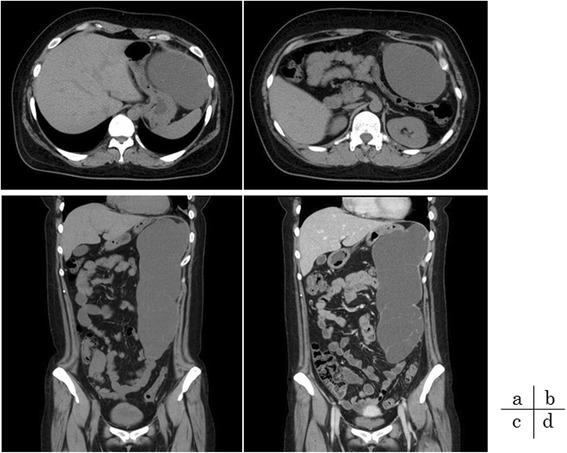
Findings of computed tomography (CT). a–c CT on the first visit to our hospital shows a multicystic tumor measuring 270 × 100 × 100 mm and continuous with the greater curvature of the stomach. d CT after 14 days of MTZ treatment reveals no change in size or structure, and no presence of nodules
